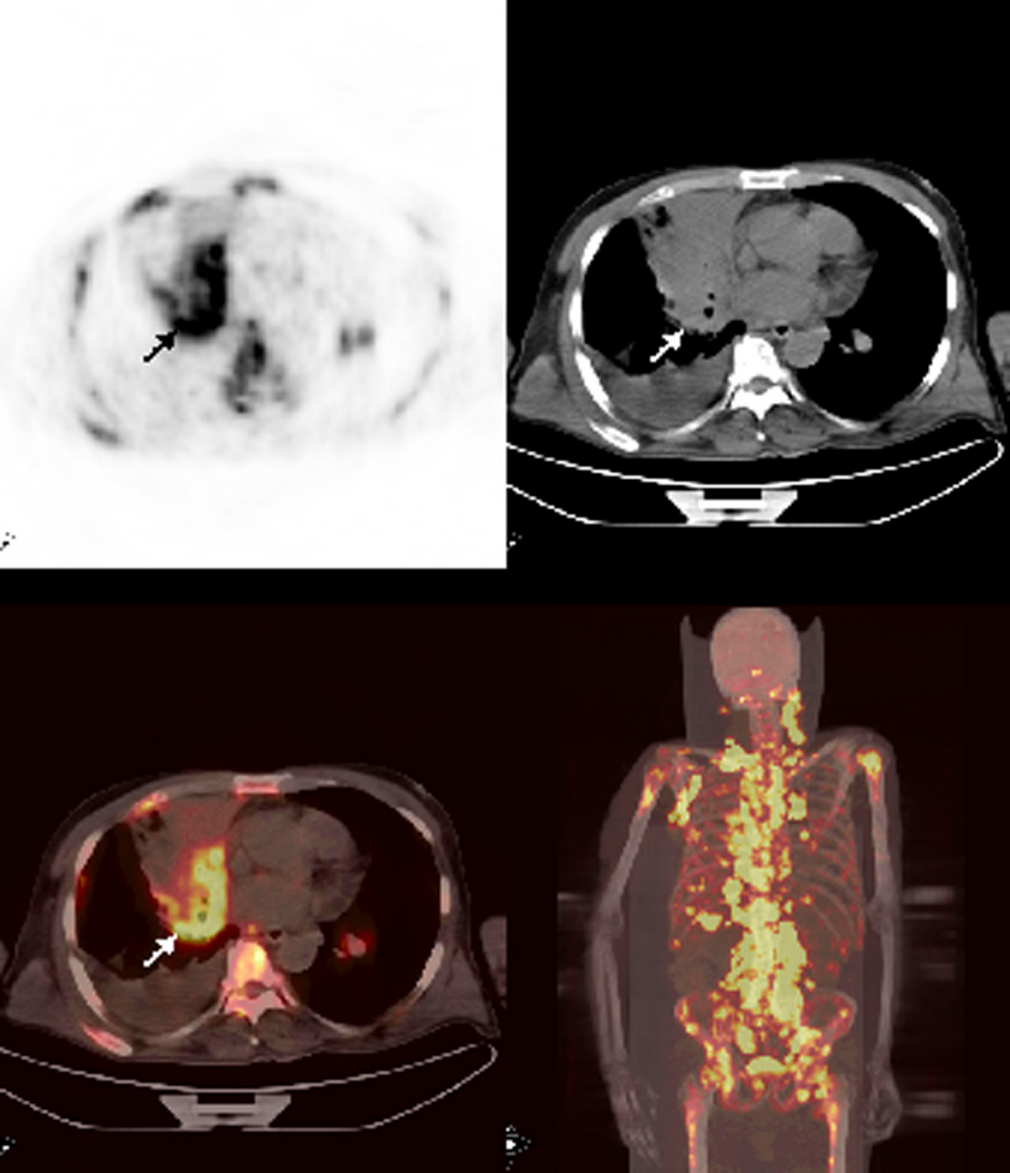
Axial and MIP PET/CT images showed a mass at the right hilar region with very intense FDG accumulation. There were also multipl bone-bone marrow metastases and extraskeletal metastases with prominent FDG uptake.
